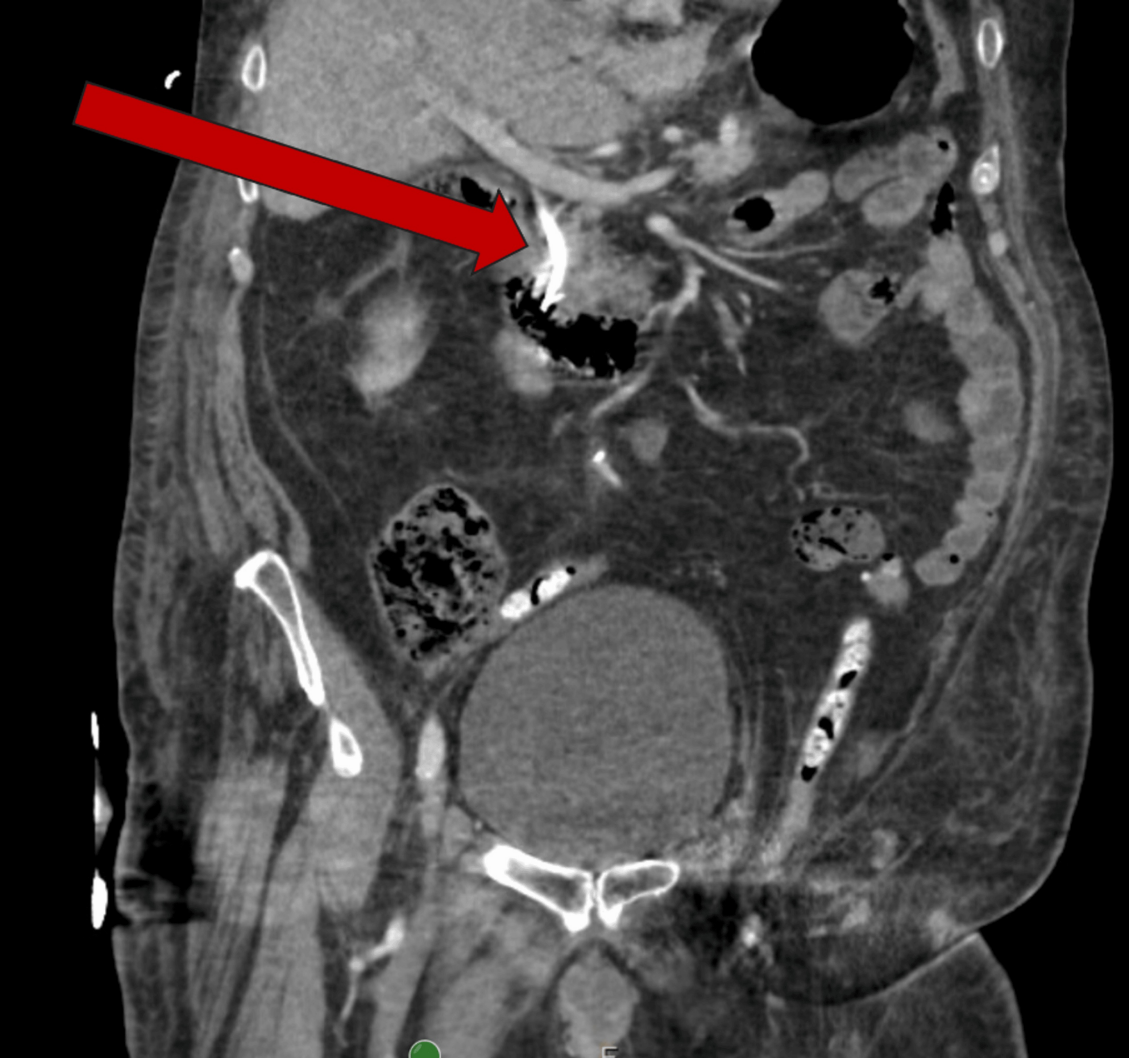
CT abdomen & pelvis showing biliary stent placement through a fistulous tract between the duodenum and common bile duct, highlighting a choledochoduodenal fistula
